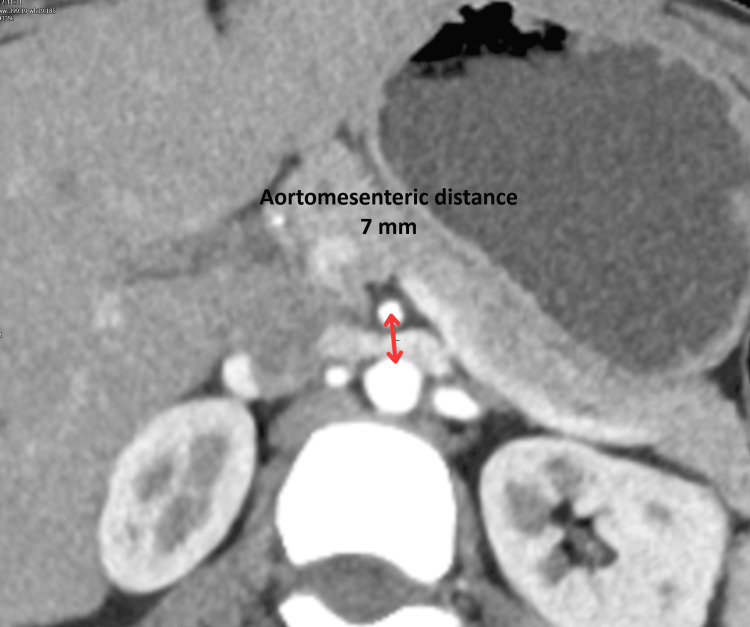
Axial contrast-enhanced CT image demonstrating reduced aortomesenteric distance (~7 mm) with compression of the third part of the duodenum between the aorta and superior mesenteric artery. CT: computed tomography
